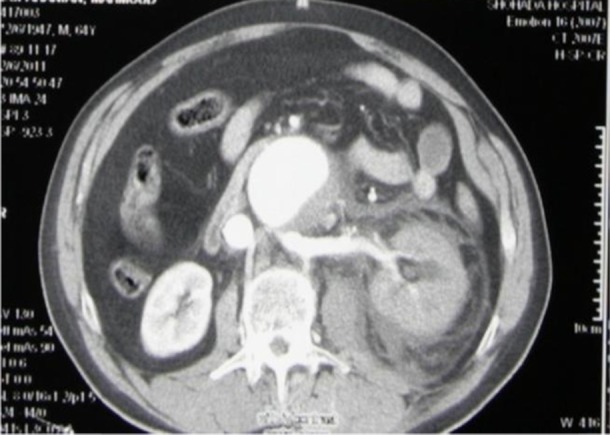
Abdominal CT of the patient showing large infrarenal aortic aneurism and fistula to IVC
